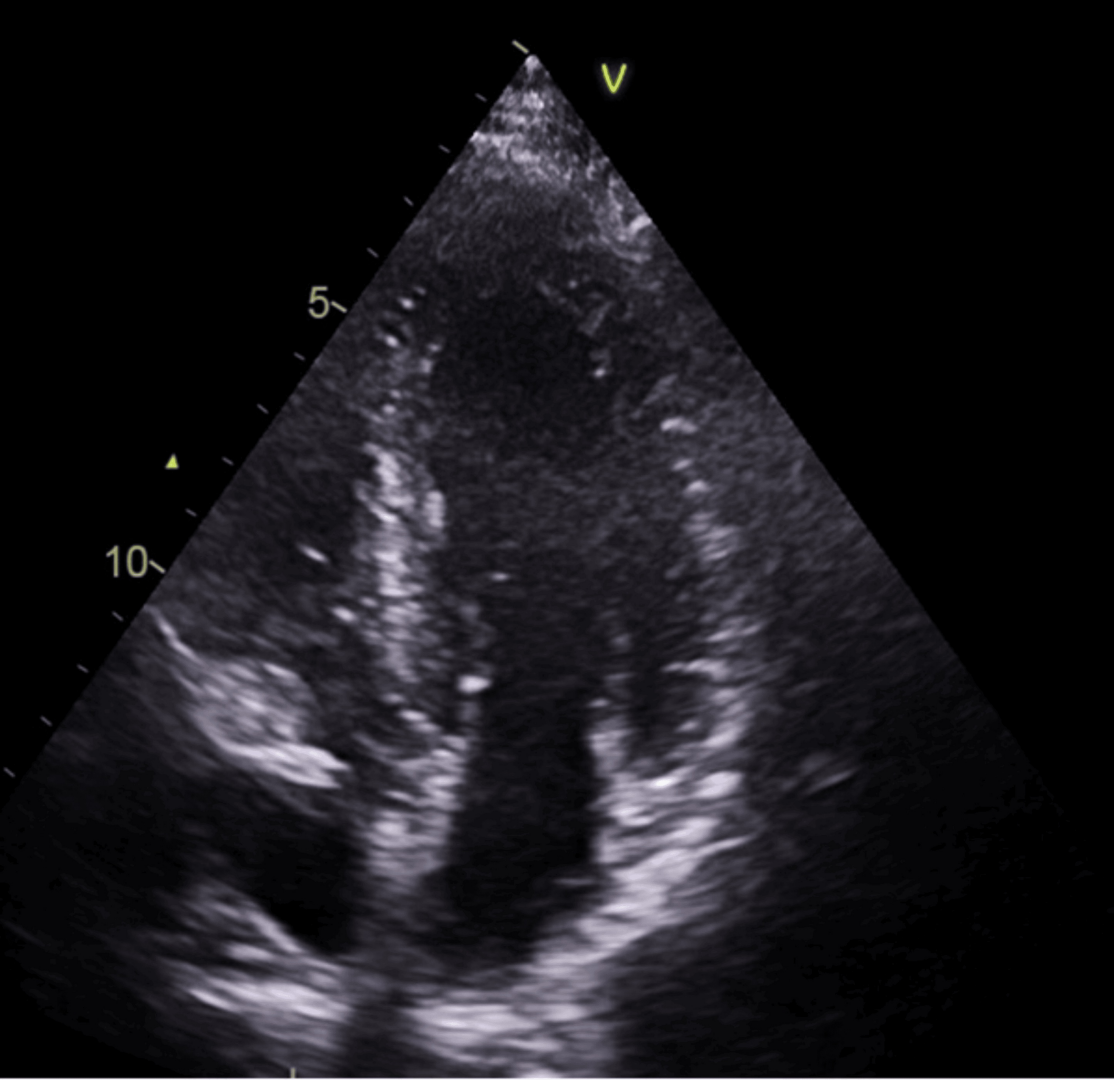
Follow-up transthoracic echocardiogram (apical four-chamber view) showing complete resolution of the previously identified LV thrombus. This image, obtained two weeks after initiation of therapeutic low-molecular-weight heparin (Clexane 1 mg/kg BID), demonstrates rapid thrombus resolution and supports the efficacy of LMWH in acute management.
